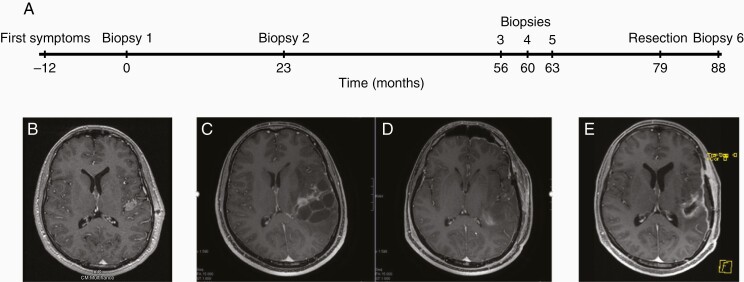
Timeline of surgical procedures and imaging results. (A) Biopsy 1 revealed an epithelioid tumor with a BRAF V600E mutation, as depicted in Figure 2 (left column). Biopsy 2 revealed a relapsing tumor with the same BRAF V600E mutation. Biopsies 3, 4, and 5 were free of tumor. The resection revealed an anaplastic PXA. Biopsy 6 was free of tumor. (B) MRI scans in March 2014, preceding biopsy 1, revealed a singular mass in the left insular cortex region (MPR sequence). (C) Pre- and (D) postoperative MRI of the recurrent tumor in September 2020 (resection; FSPGR sequence). (E) In January 2021, MPR sequence was performed 6 weeks after radiotherapy (3 months after the resection; not shown in the timeline). Abbreviations: FSPGR, fast spoiled gradient echo; MPR, multiplanar reformation; MRI, magnetic resonance imaging; PXA, pleomorphic xanthoastrocytoma.
